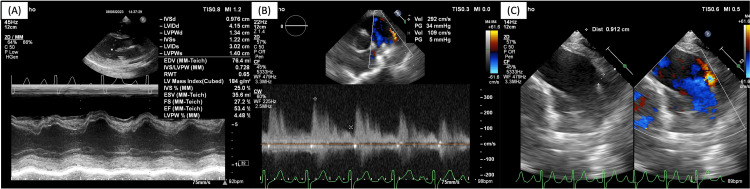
Pre-procedural transthoracic and transoesophageal echocardiography. Echocardiography showing (A) LV concentric hypertrophy with normal LV systolic function, (B) continuous left-to-right ductal flow, and (C) PDA diameter of 9 mm. LV, left ventricle; PDA, patent ductus arteriosus.
